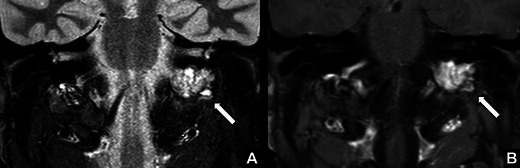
Glomus jugulare tumour.*A 42-year-old man presenting with slowly progressive symptoms of left VCP with no other apparent laryngeal pathology. a Coronal T2-weighted MRI images and b T1-weighted contrast-enhanced fat-saturated images showed a T2 hyperintense avidly enhancing, smooth lobulated mass, with a salt and pepper appearance at the left jugular foramen (*arrows*) typical of glomus jugulare tumour
